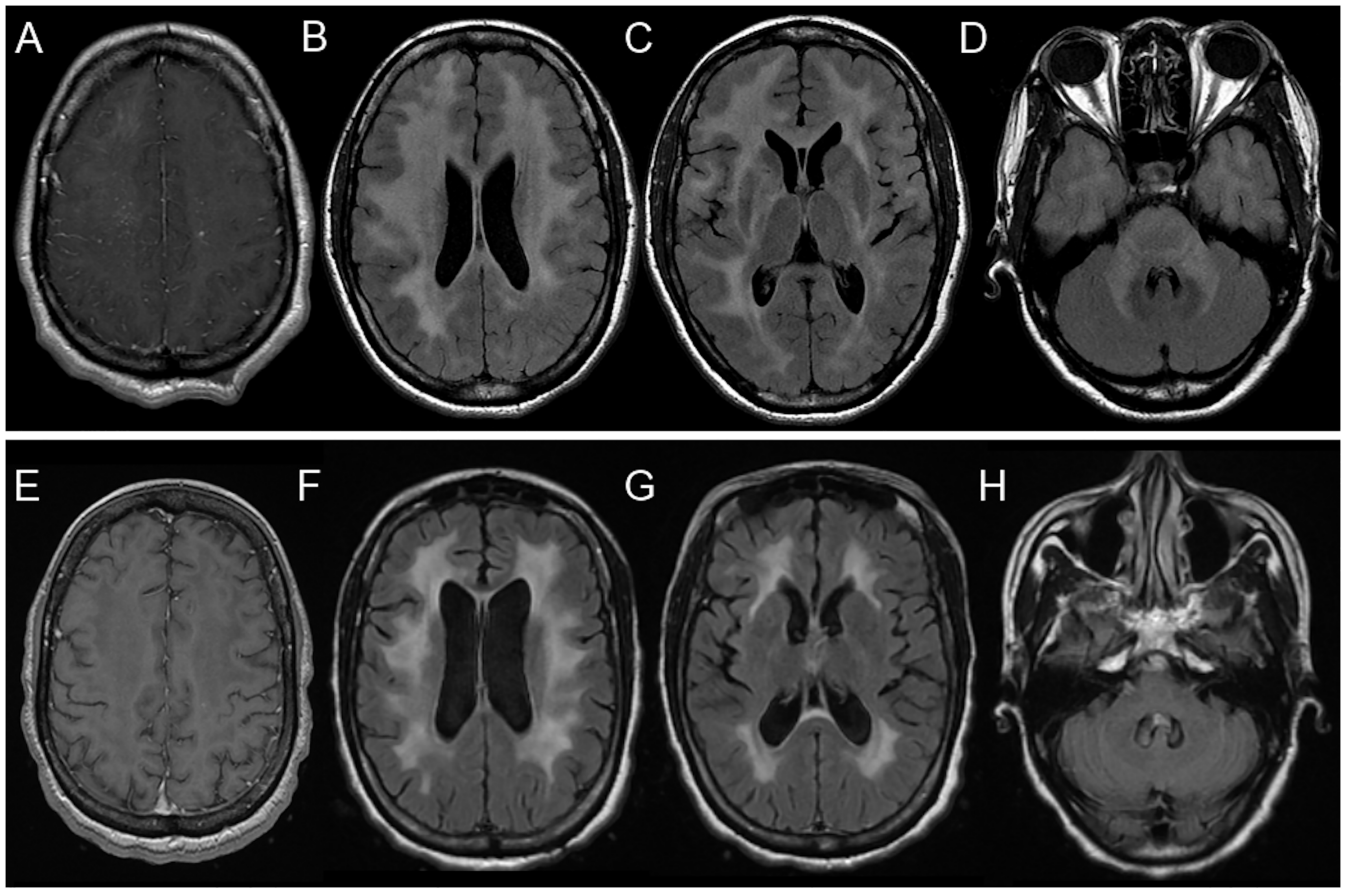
Brain MRIs of the mother before and after treatment of the meningoencephalitic stage of trypanosomiasis. (A) Initial brain MRI in January 2013 (axial T1-weighted image after injection of gadolinium chelates) showing perivascular and leptomeningeal enhancement, predominantly in the right corona radiata. (B, C, D) Initial brain MRI in January 2013 (axial fluid attenuated inversion recovery [FLAIR]-weighted images) showing extensive and confluent hyperintensities in both corona radiata and bilateral fronto-parieto-occipito-temporal white matter, internal and external capsules, lenticular nuclei, metencephalon, and middle cerebellar peduncles. Global brain atrophy with enlarged cerebrospinal fluid (CSF) spaces for a 22-year-old patient. (E) Control brain MRI in June 2014, 1 year after treatment (3D-T1 weighted image after injection of gadolinium chelates), showing resolution of the perivascular and leptomeningeal enhancement. (F, G, H) Control brain MRI in June 2014, 1 year after treatment (axial FLAIR-weighted images), showing resolution of the infratentorial and basal ganglia lesions, diminution of the supratentorial white matter lesions (especially capsular lesions), and increased secondary atrophy.
